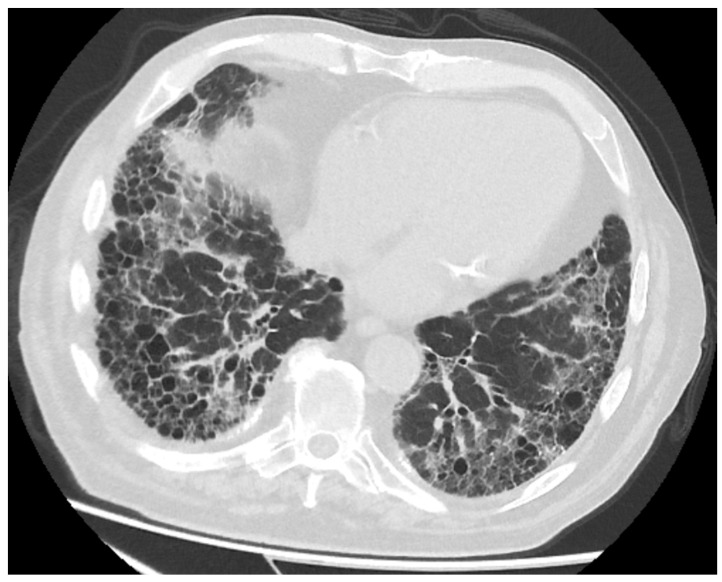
Computed tomography showing interstitial fibrosis.
